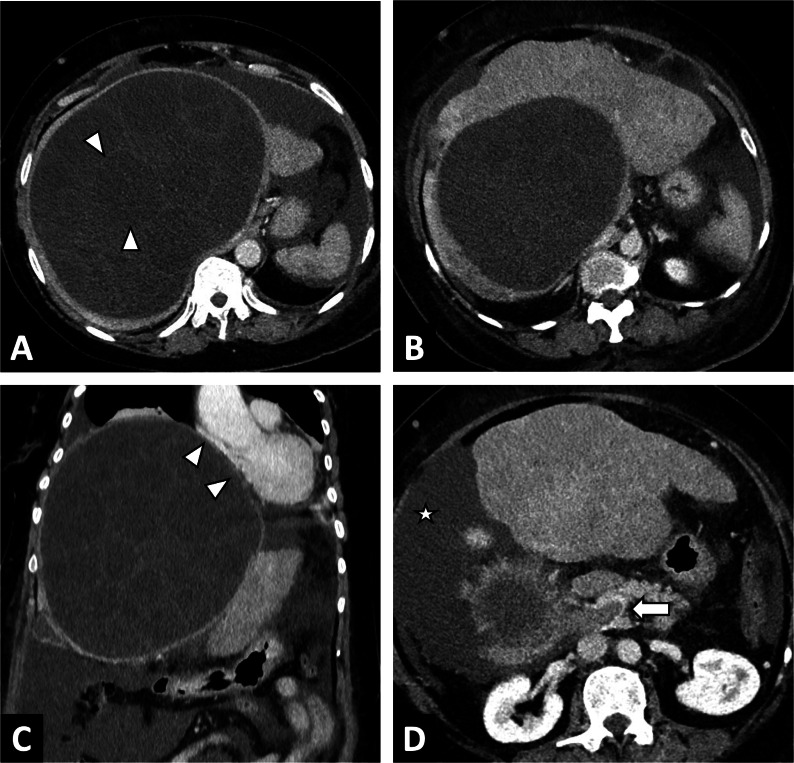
Large cystic echinococcosis lesion of the right liver in a 54-year-old woman revealed by ascitic decompensation. A CT image in the axial plane at the portal phase showing a large cystic echnicoccosis lesion of the right liver. Internal daughter cysts (arrowheads) (CE3a stage). B CT image in the axial plane at the portal phase showing a large right liver cyst complicated by advanced chronic liver disease secondary to Budd Chiari syndrome. Hypertrophy of the left liver lobe and surface nodularity suggestive of advanced fibrosis. C Same examination in the coronal plane. Mass effect of the cystic echinococcosis on the heart chambers (arrowheads). D Same examination in the axial plane, lower slices. Portal thrombosis (arrow) and ascites (star)
